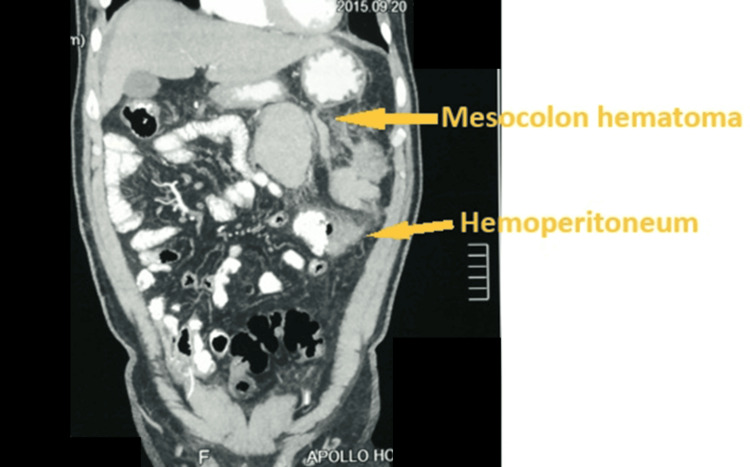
Coronal CT image showing mesocolon hematoma with hemoperitoneum
